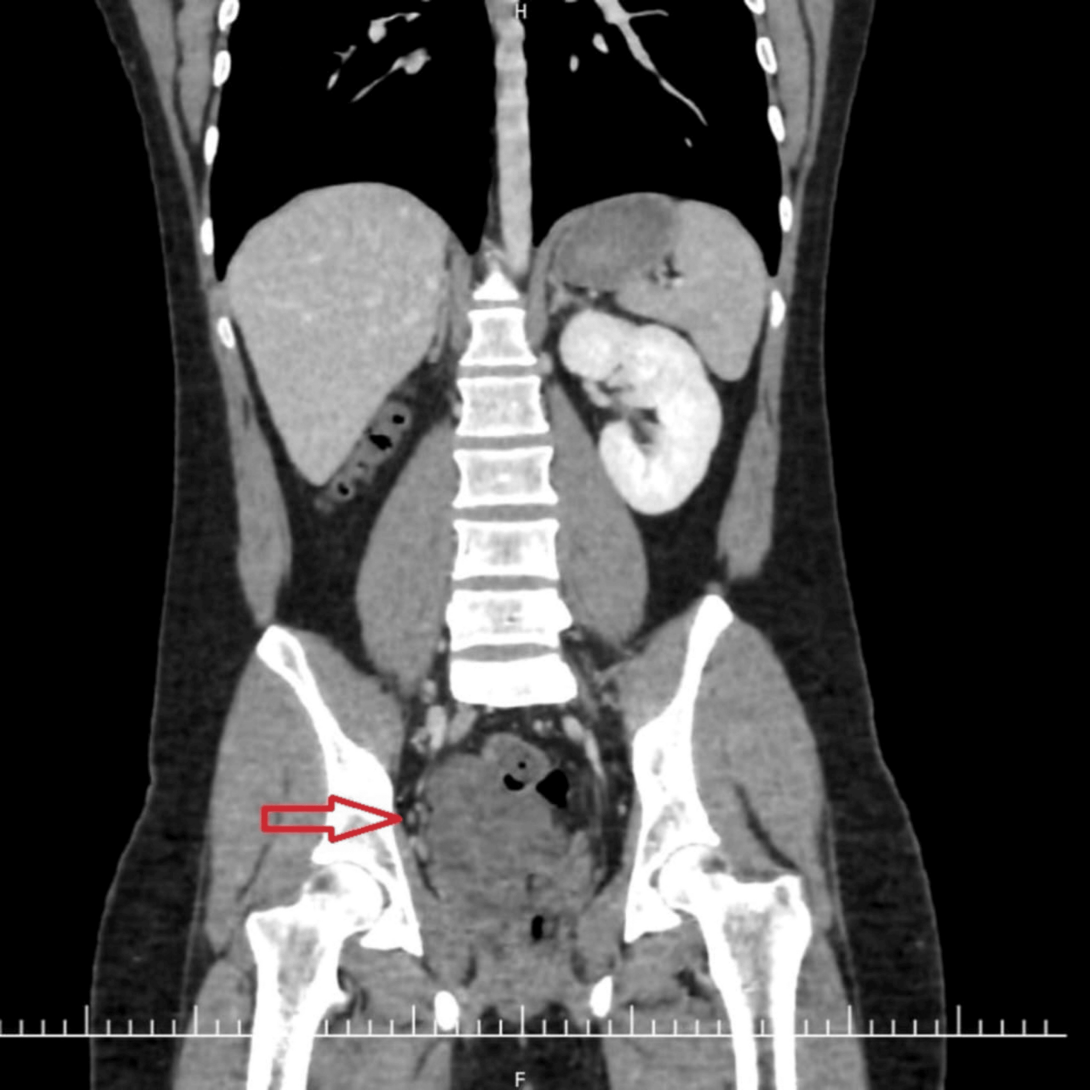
Coronal contrast-enhanced CT urography image shows complete absence of the right kidney and a large, well-defined, septated cystic lesion in the right paravesical space, suggestive of a seminal vesicle cyst (arrow). CT, Computed tomography
